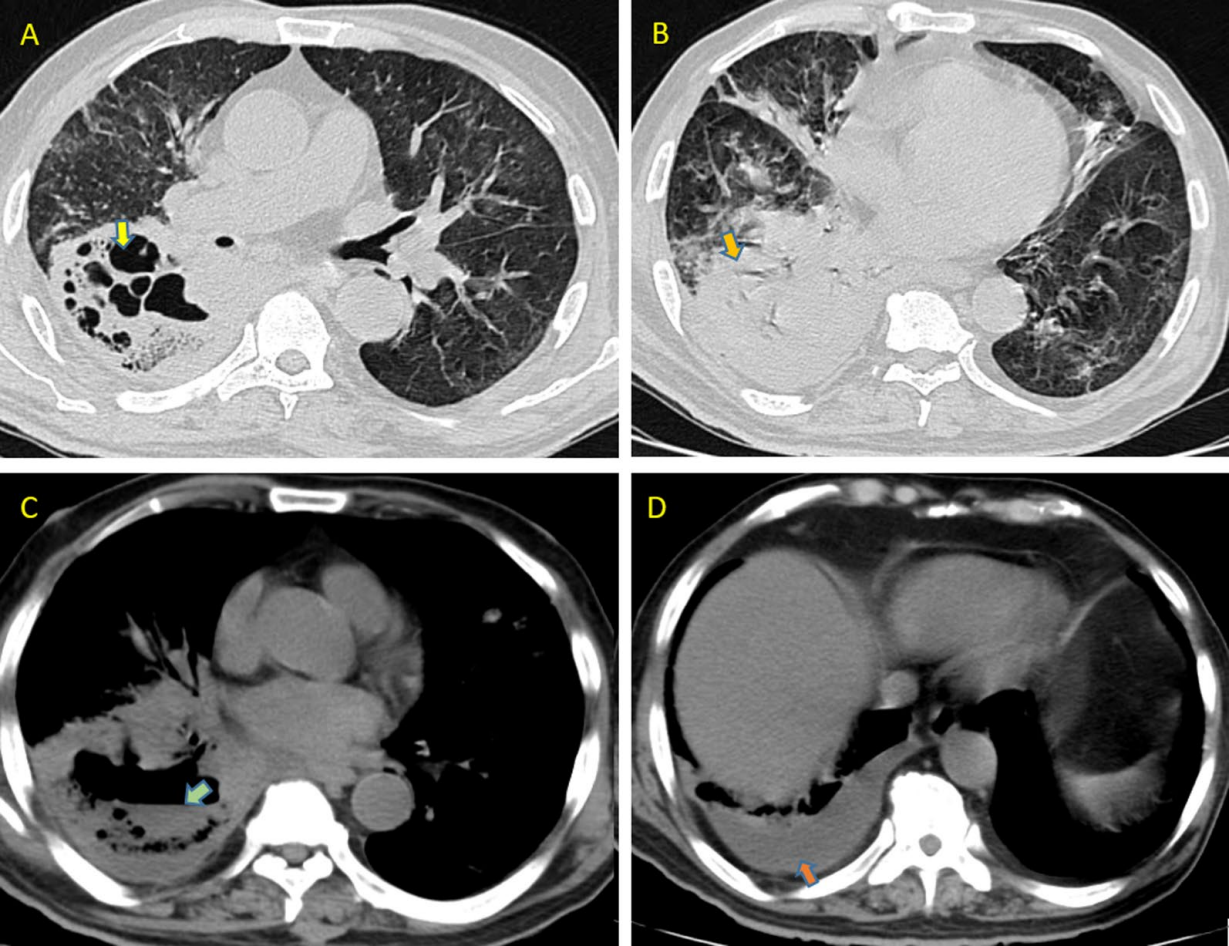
Axial HRCT scans showing imaging features of Mucormycosis in Case 4 of COVID-19. 4 A, B CT axial image large cavitary lesions (yellow arrow in A) with thick internal septations, surrounded by thick consolidation. Dense consolidation also noted in left lower lobe (orange arrow in B). C Air fluid level within cavity (green arrow in C). D Minimal right pleural effusion (dark orange arrow in D)
